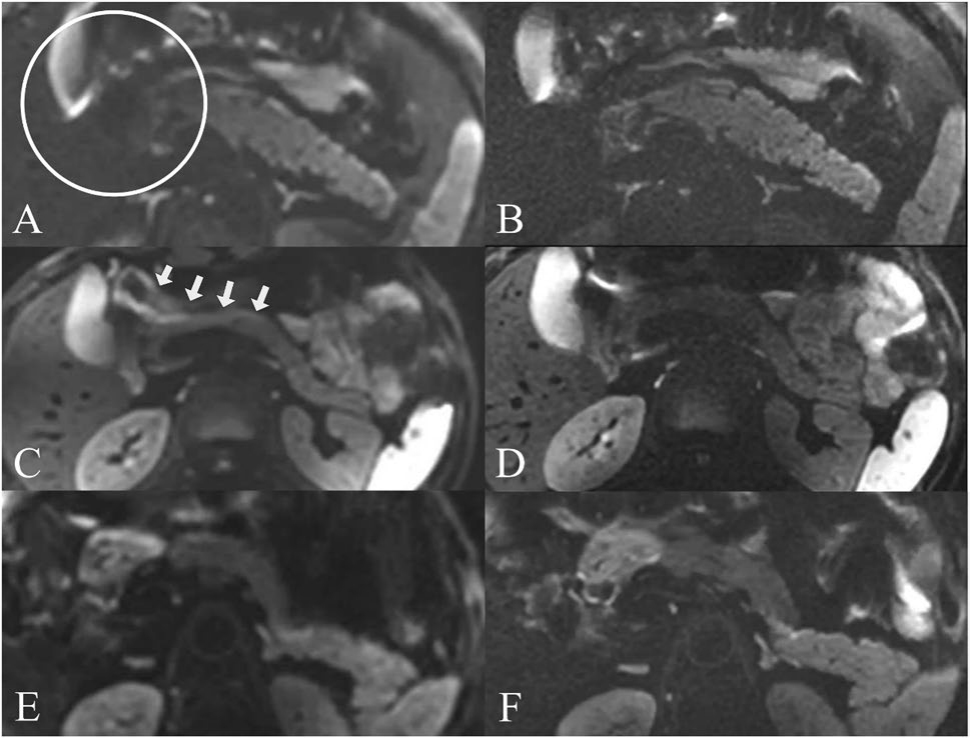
b800 of lFOV (A, C, E), b800 of rFOV (B, D, F). According to the image, rFOV images have a greater resolution and more clear contours than lFOV images. In A, B, on the b800 DWI image of lFOV, as depicted by the white solid circle, the head of the pancreas is obscured by the adjacent gallbladder and surrounding intestines, and the partial display is not clear. In C, D, the position shown by the white pointed head, the pancreas appears pseudo-diffusion-restricted in lFOV due to the convolution effect of the surrounding intestine, this effect disappears in rFOV DWI. In E, F, demonstrate that the diffuse look of the body and tail of the pancreas on DWI images is distinct, because of that the head, body, and tail of the pancreas have distinct cellular compositions.
